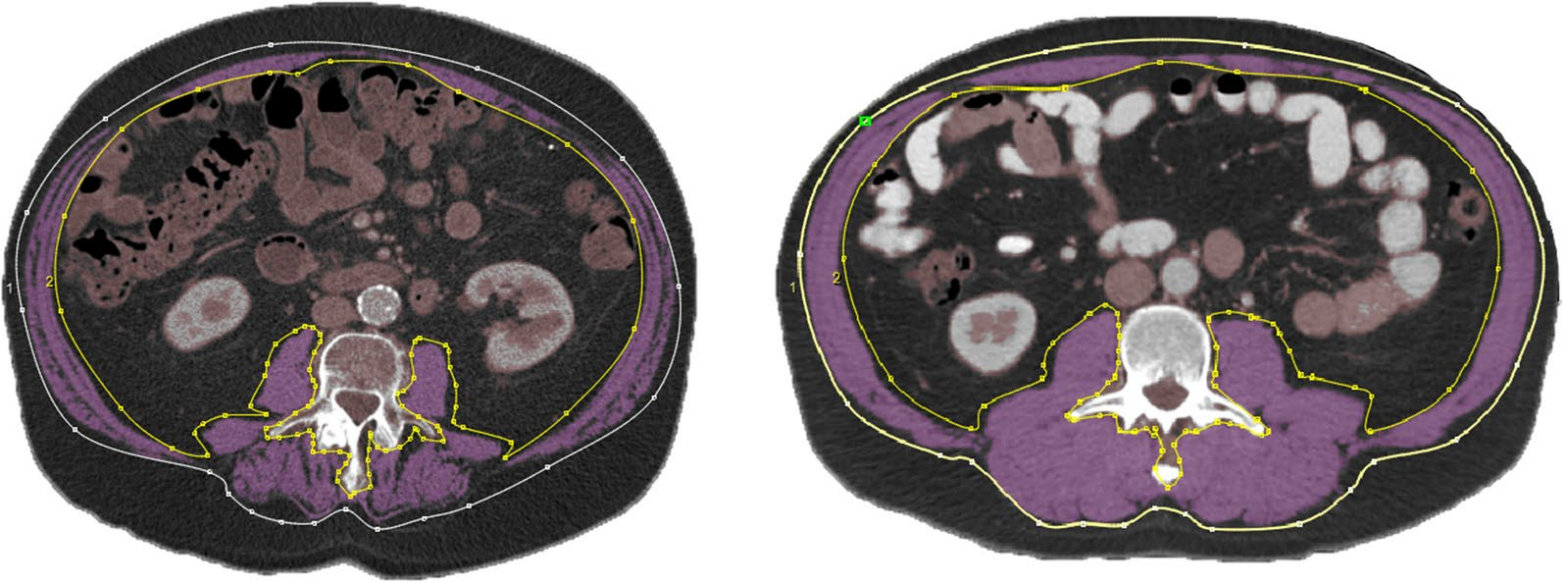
CT scans at the third lumbar vertebral level of a patient with sarcopenia (left) and a patient with normal skeletal muscle mass and density (right). The skeletal muscles are outlined
